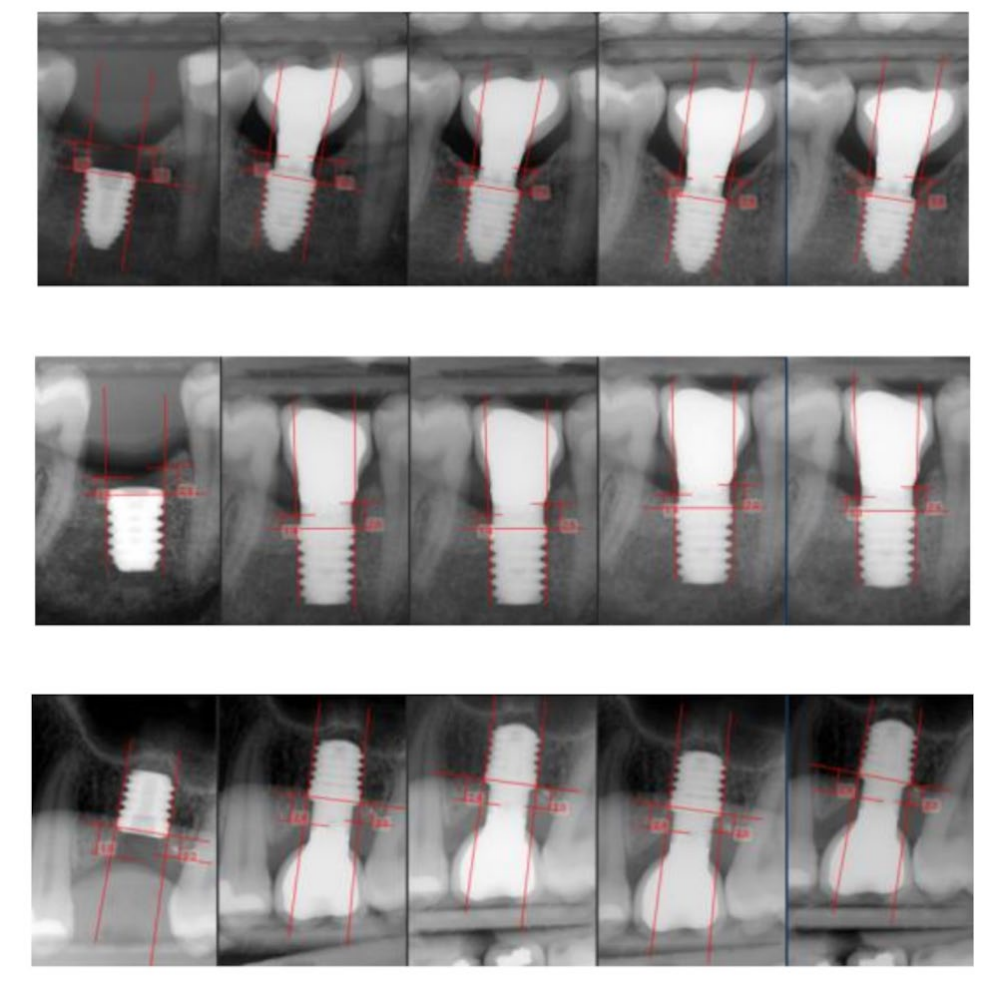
Radiographic images with follow-up for the 3 heights (1.5, 2 and 3 mm) at the 5 control points: implant surgery, crown placement, 3 months, 6 months and 12 months after loading
